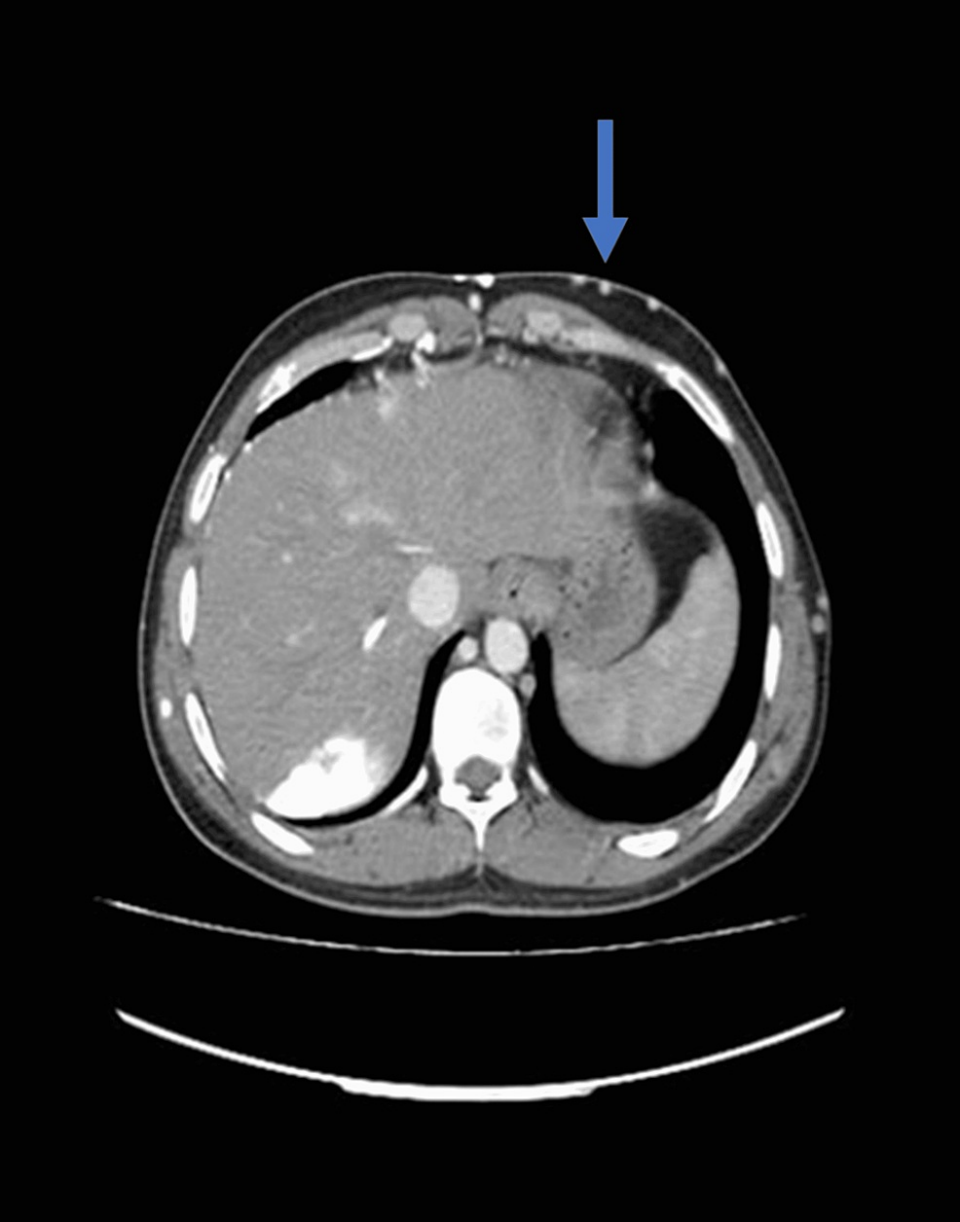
CT scan abdomen showing abdominal wall collaterals CT: computed tomography
